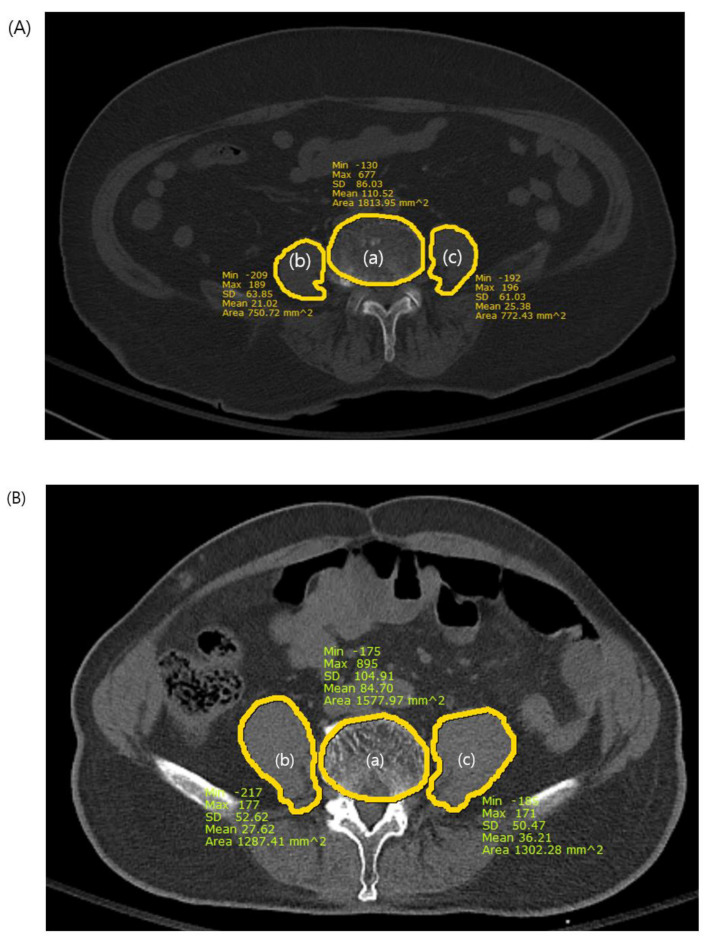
The psoas-lumbar vertebral index (PLVI) measurement. (a) L4 vertebral body area, (b) right psoas area, (c) left psoas area. PLVI = [(b + c)/2]/a (A): PLVI of the sarcopenic patient on computed tomography. The PLVI= 0.41. (B): PLVI of the nonsarcopenic patient on computed tomography. The PLVI = 0.84.
